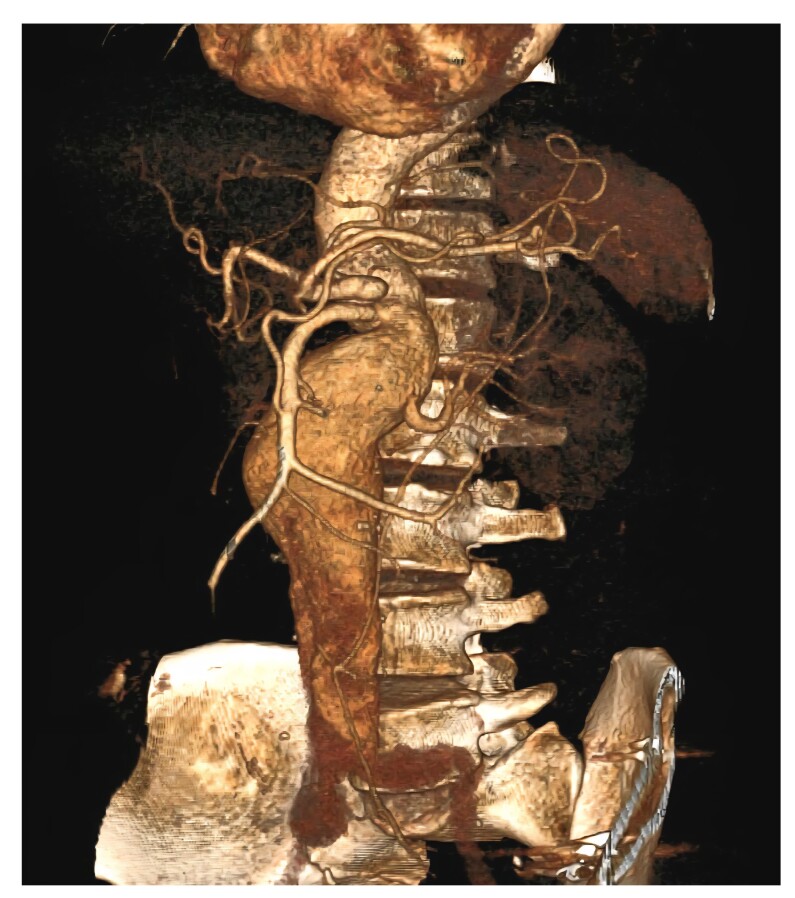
Preoperative 3D reconstructed CT angiography showing a fusiform dilatation of the abdominal aorta from L2 to L5 vertebral level with the left renal artery arising from the aneurysmal segment (suprarenal abdominal aortic aneurysm).
